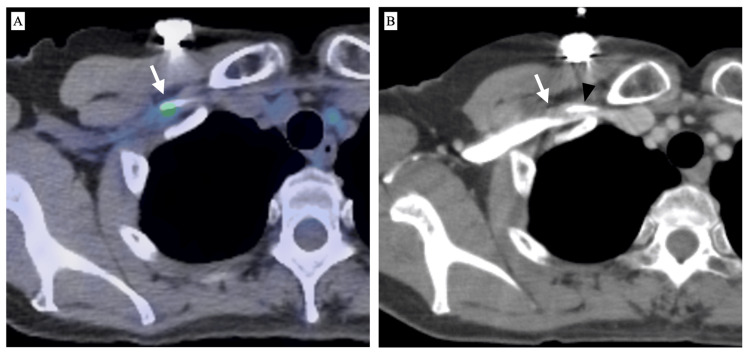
FDG-PET/CT and CE-CT scans of a 59-year-old woman with malignant lymphoma A: FDG-PET/CT 167 days after TIVAD implantation shows a subtle accumulation in the subclavian vein (arrow); B: CE-CT shows a small thrombus (arrow) around the catheter (arrowhead) FDG: Fluorodeoxyglucose, TIVAD: Totally implantable venous access device
